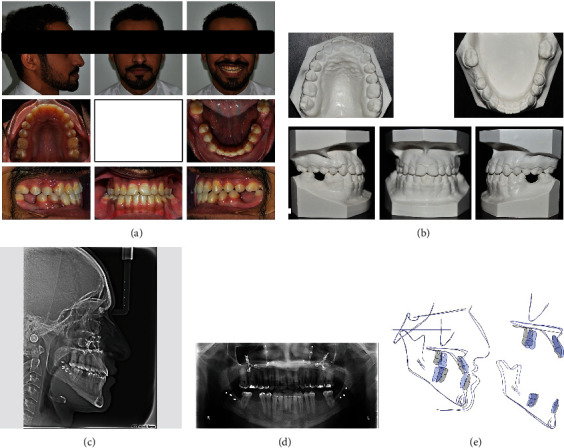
Posttreatment extraoral and intraoral photographs, study models cephalometric radiograph, and panoramic radiographs of the patient: (a) extraoral and intraoral photographs; (b) study models; (c) cephalometric radiograph; (d) panoramic radiograph; (e) cephalometric superimposition.
